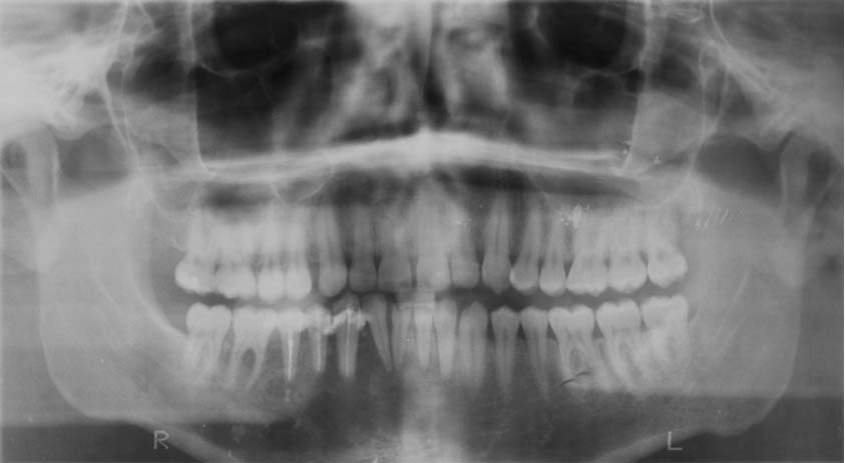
Panoramic radiograph, taken two weeks before presentation by the attending dentist, showing an osteolytic process in the right mandible and the teeth 43–45 after root channel treatment and root amputation.
